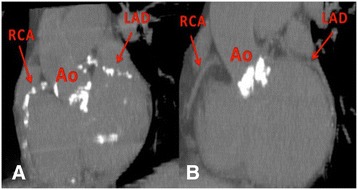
Demonstration of typical noncontrast CT study of a patient with severe calcified aortic stenosis and extensive difuse coronary calcifications (picture A) in contrast with patient without concomitant coronary disease (picture B).
